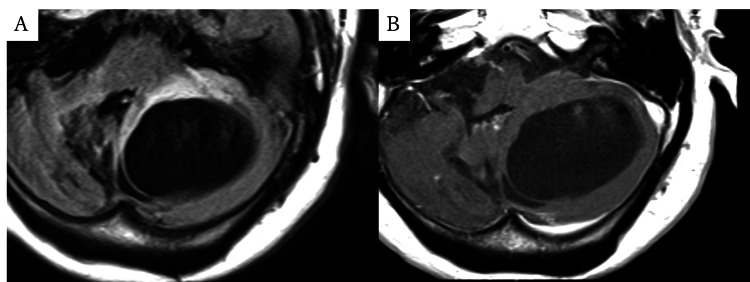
Preoperative MRI (A) FLAIR image showing the cystic lesion isointense to CSF. (B) Contrast-enhanced T1-weighted image showing no enhancement.
